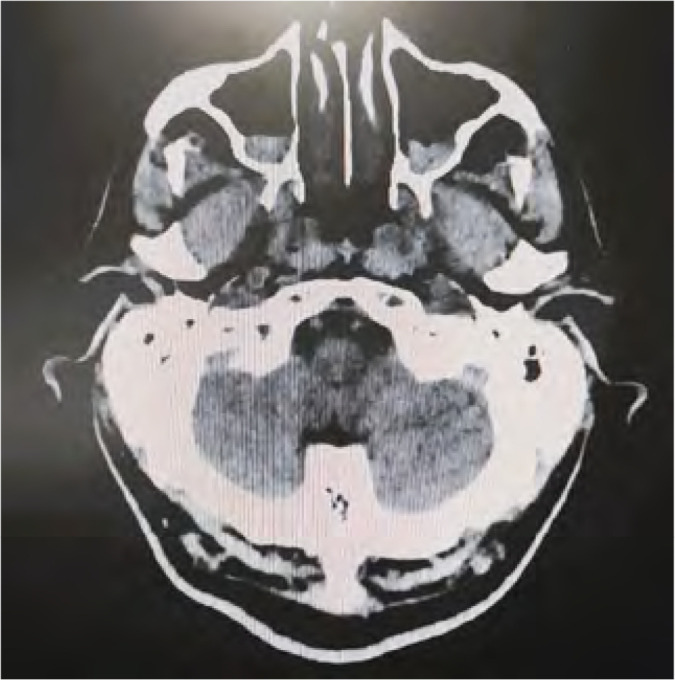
CT of the brain revealed pansinusitis.
